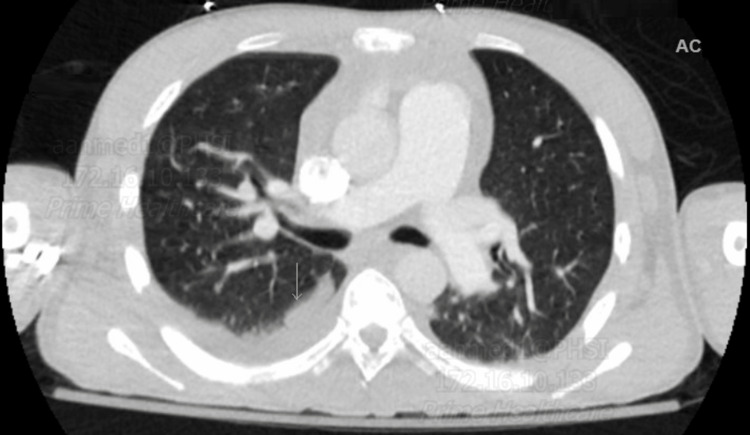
CTA chest axial lung window showing right lower pleural effusion with possible atelectasis and consolidation. CTA: CT angiography
